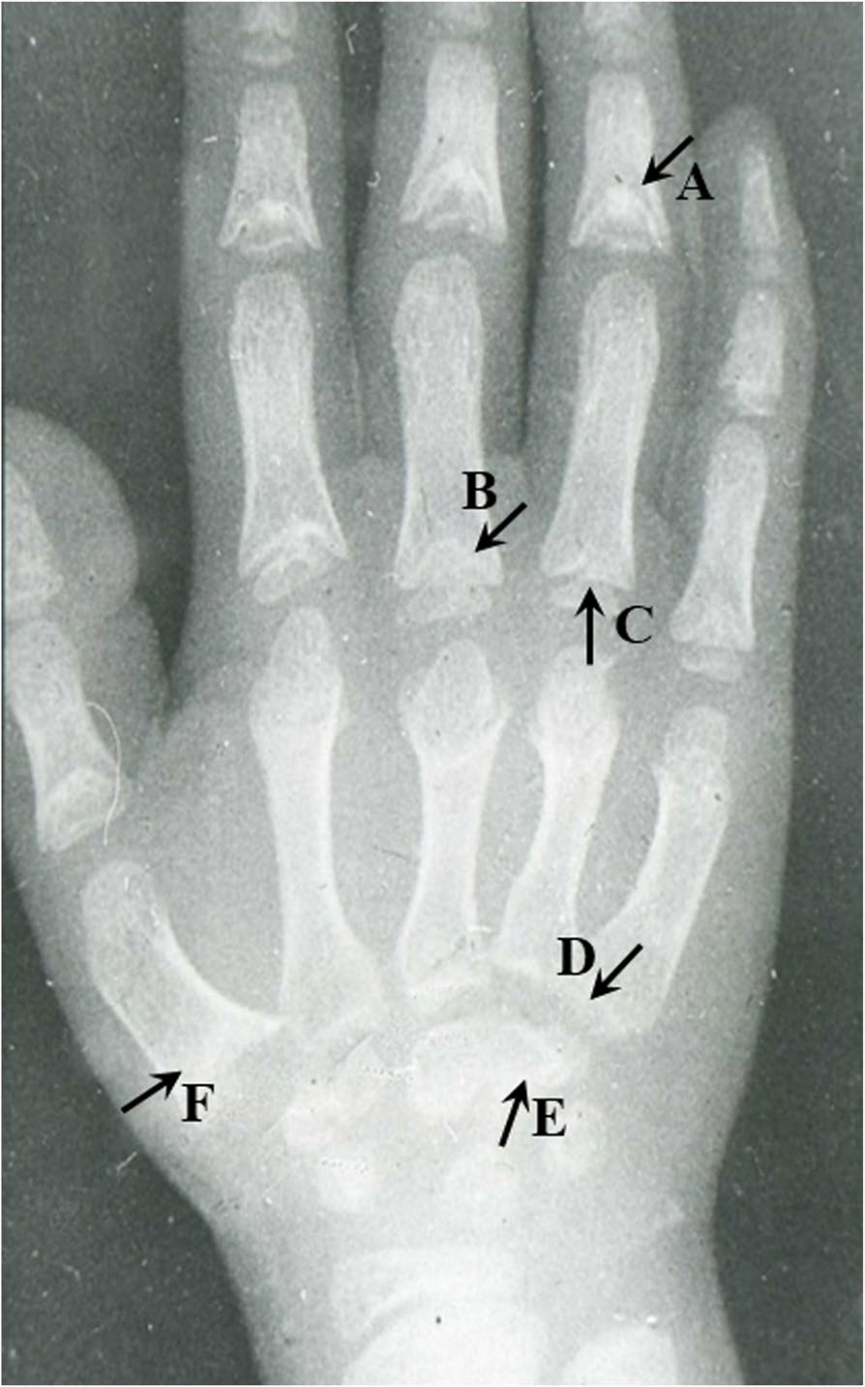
Examples of X-ray pathological changes of an eight-year old KBD boy. A A large defect with cone shaped showed in metaphysis alterations. There is an early closure of epiphysis line; B A large defect in metaphysis; C Cone shaped epiphysis; D Sclerosis in bottom of metacarpal bone; E Irregular marginal with sclerosis in carpal; F A large defect with sclerosis in carpal
